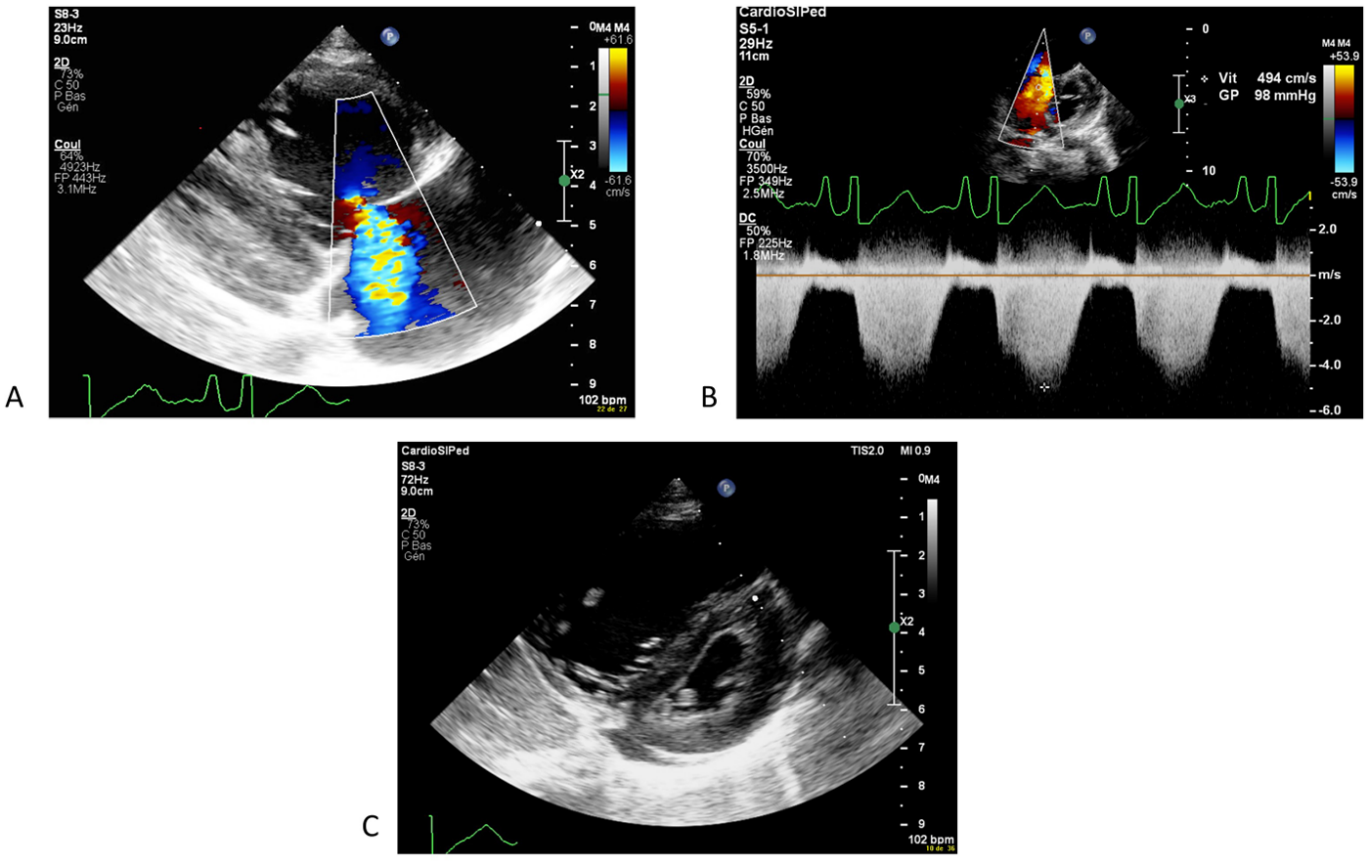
First echocardiography showing a severe pulmonary hypertension with tricupsid regurgitation (A) allowing a measure of right ventricular pressure of 98 mmHg on continous dopplrd (B) and a flattened interventricular septum (C).
